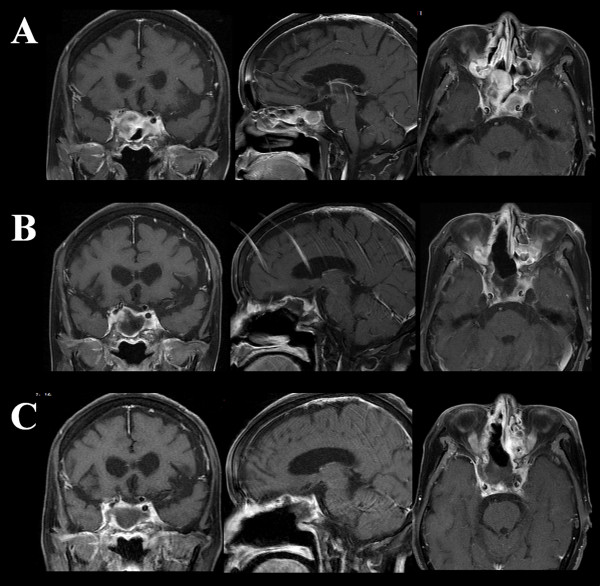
After chemotherapy and cyber-knife treatment. A. Tumor regrowth is noted after discontinuation of ICE chemotherapy on MRI. B. The cavernous sinus portion of the tumor is noted after the third tumor resection. C. No obvious tumor recurrence is identified seven months after cyber-knife radiation.
